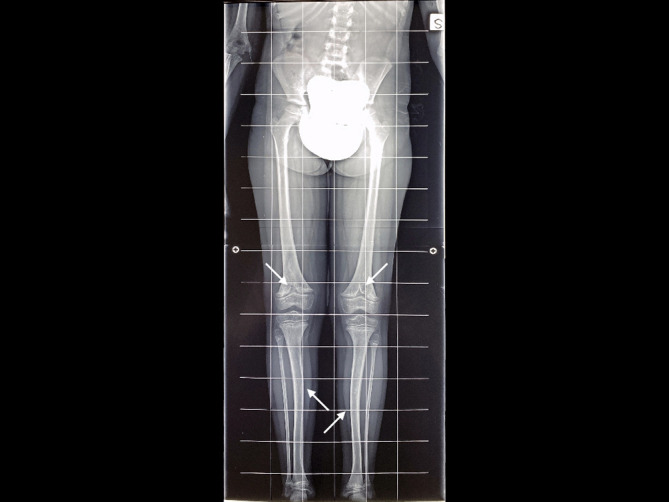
Radiographic findings in the elder sister (Family 1). Imaging reveals tibial deformities and metaphyseal irregularities, consistent with hypomineralization, despite the absence of overt clinical symptoms.
